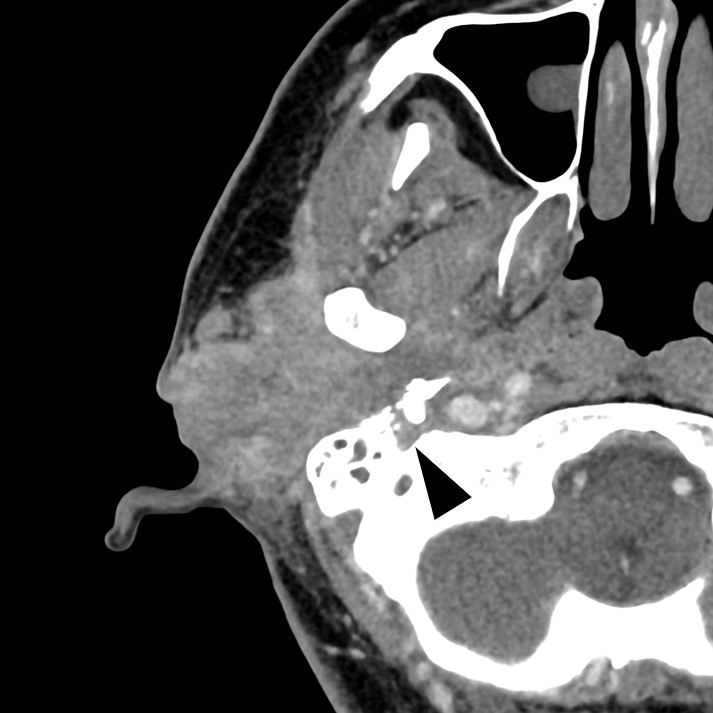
Contrast-enhanced computed tomography shows a contrast-enhanced tumor extending from the right external auditory canal to the piriformis and invading the right facial nerve. The arrowhead indicates the tumor extending into the stylomastoid foramen.
